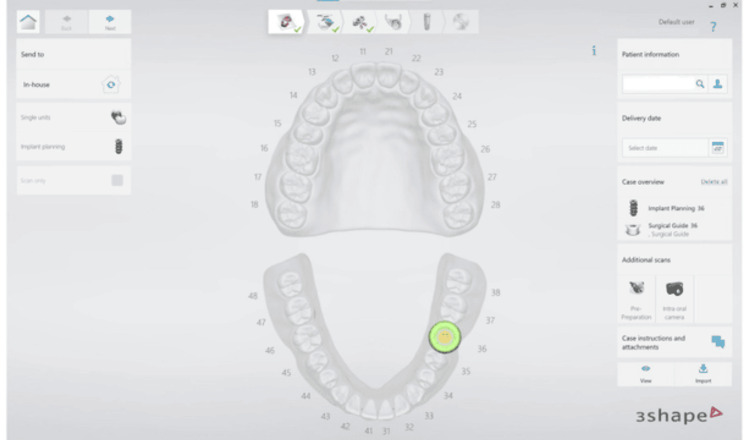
Importing CBCT and STL files into 3Shape Implant Studio for implant planning and surgical guide design CBCT: cone beam computed tomography; STL: Standard Tessellation Language
